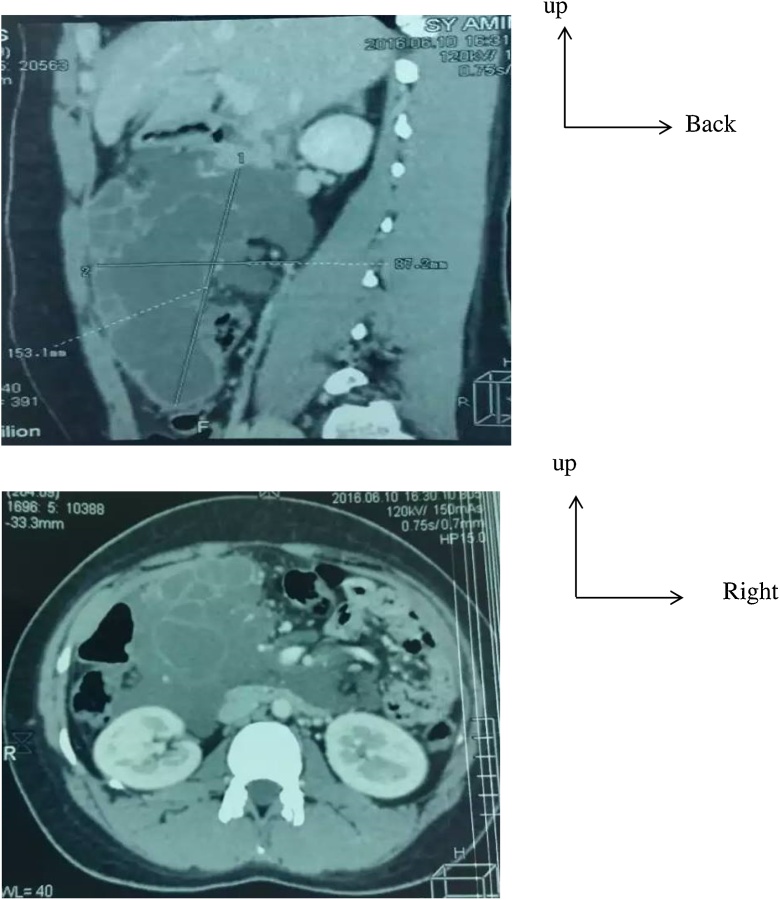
Abdominal CT scan with contrast showed cyst mass mesured 15 cm * 6,3 cm, subhepatic, with fluid density (arrow) encompassing multiples mesenteric vessels.
